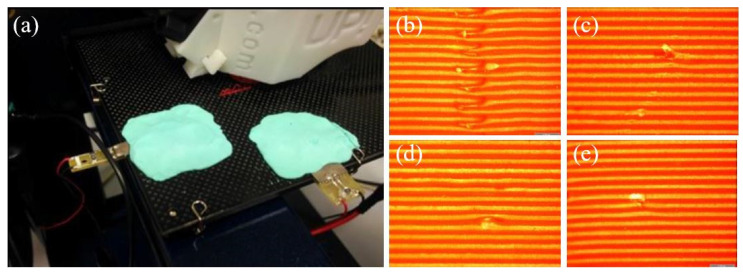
(a) Ultrasonic transducers mounted to the printing platform. (b) Surface of the original ABS sample. (c) The 11 kHz treated surface. (d) The 16 kHz treated surface. (e) The 21 kHz treated surface. The quality of the surface finish improves with the increment of the frequency [255].
